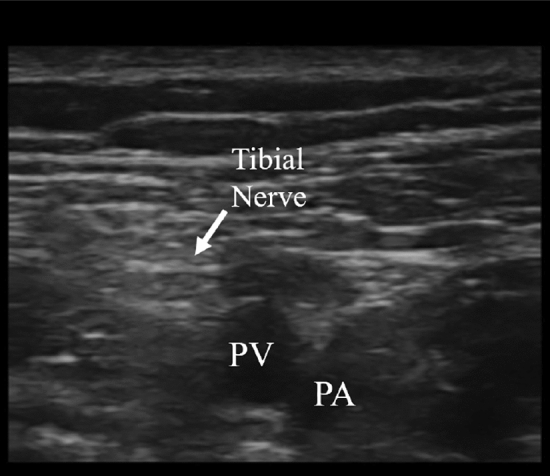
Ultrasound image for finding the tibial nerve. PA: Peroneal artery, PV: Peroneal vein.
